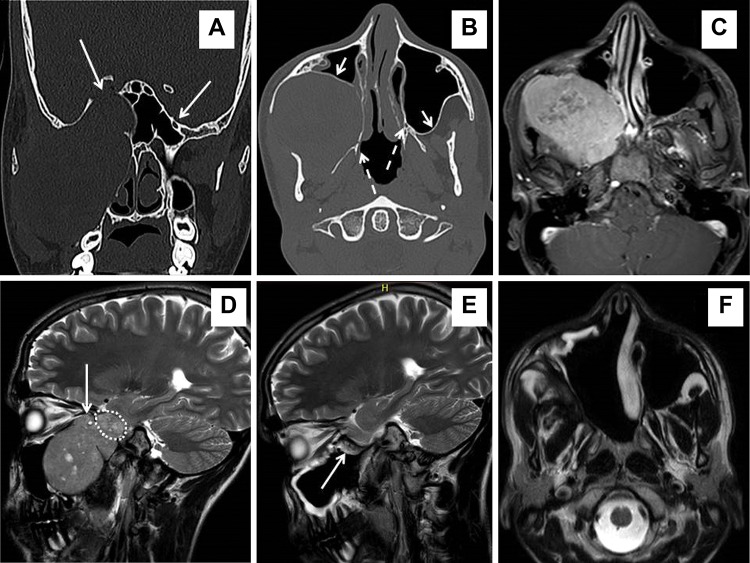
Schwannoma of V2, case P11. Coronal (A) and axial CT (B) scans showing considerable deformation of the splanchno and neurocranium on the right side. A mass (74 x 65 x 61 mm) arising from V2 in the pterygopalatine fossa with bulky extension to the ITF, cheek, and middle cranial fossa via the foramen rotundum, which is extremely enlarged *(long arrow)* – see side difference. The schwannoma pushed the posterior wall of the maxillary sinus forward *(short arrow)* and the pterygoid process backward *(dashed arrow)* – also see side differences. (C) An axial T1-weighted MRI scan with gadolinium contrast showing the margin between the tumor and soft tissue. (D) A sagittal T2-weighted MRI scan showing that the tumor is pressing on the orbital apex via the infraorbital fissure *(long arrow)* with an intracranial portion (24 x 21 x 20 mm) in the middle cranial fossa *(dotted line)*. Postoperative T2-weighted MRI scans in the sagittal (E) and axial (F) planes demonstrate no evidence of disease 21 months after surgery. A nasoseptal flap *(long arrow)* was used to cover exposed dura mater of the middle cranial fossa, although no clear leakage of cerebrospinal fluid was observed intraoperatively. Considerable retraction of soft tissue into the postresection empty space of the cheek and ITF is visible on the axial MRI – compare (C) and (F).
